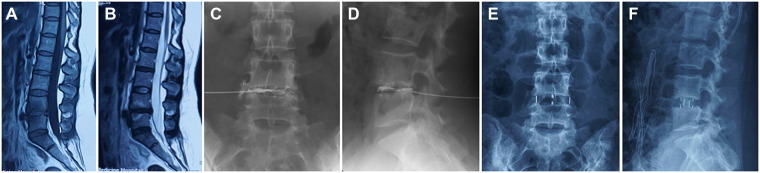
Typical case. Type I Modic changes was characterized by low T1 and high T2 signals in MRI on L4-5 endplate (A,B). The patient experienced severe LBP and DBP was a definitive diagnosis by discography and discoblock (C,D). The patient underwent OLIF stand-alone (E,F).
